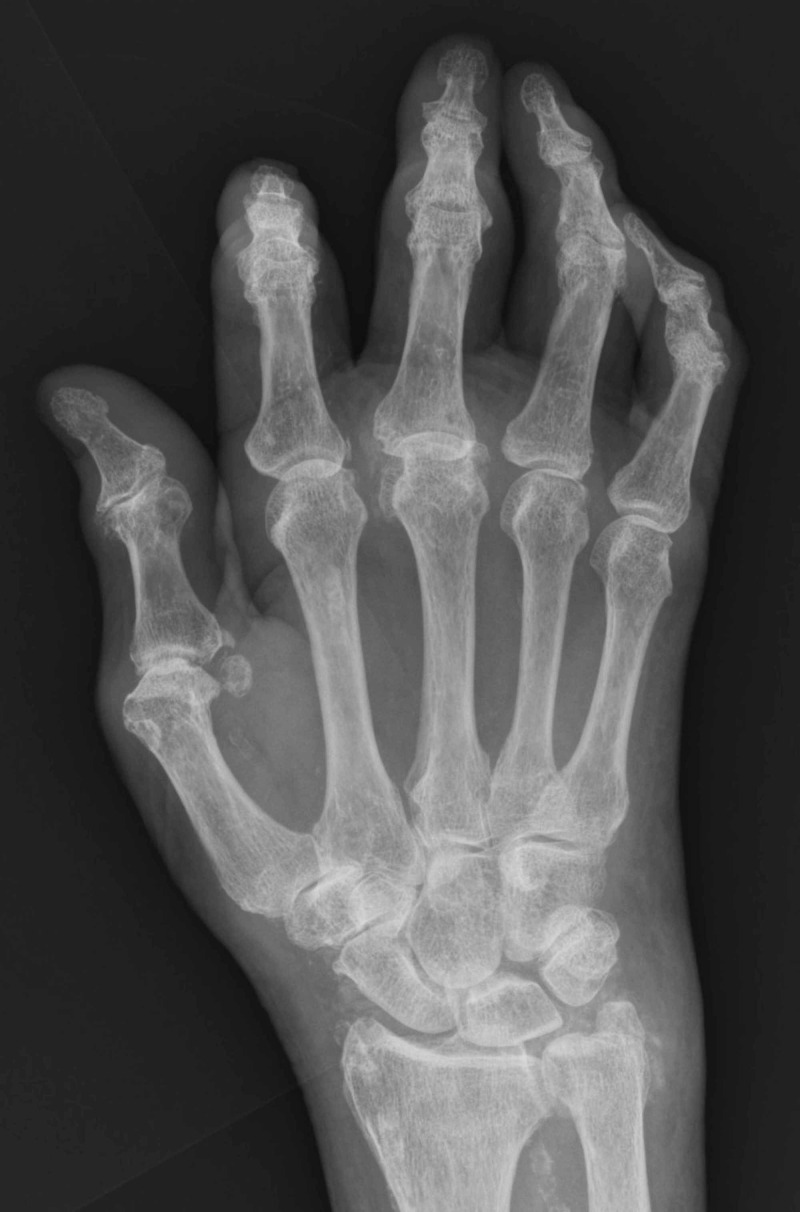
Radiography of the right hand. No bone involvement is observed.
